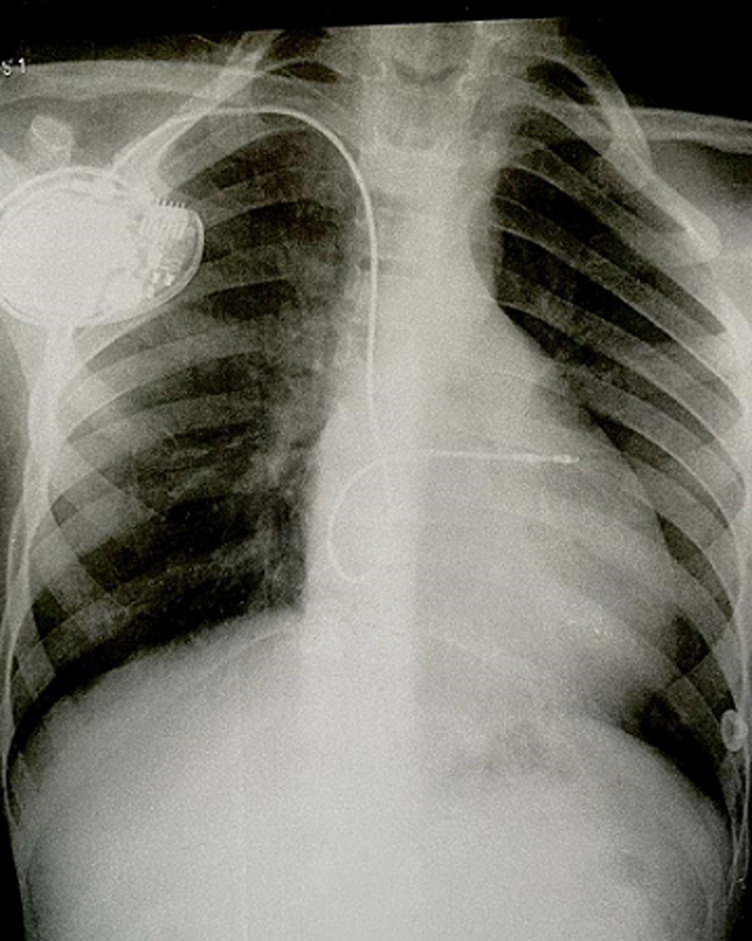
pacemaker implantation on chest X-ray
